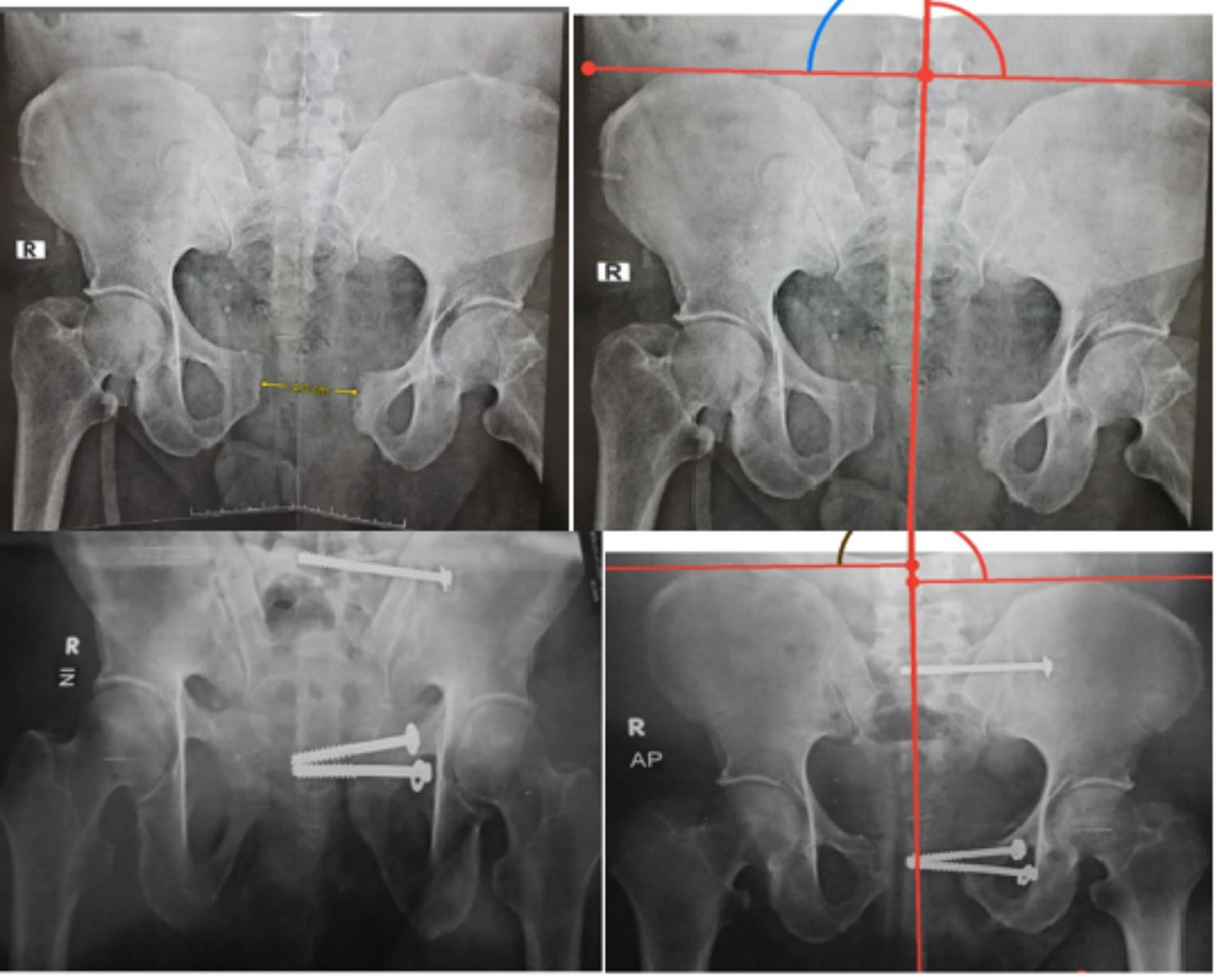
Preoperative and last follow up plain radiographs showing a failed case of symphyseal screw fixation with measurement of vertical pelvic displacement using Henderson horizontal reference lines
